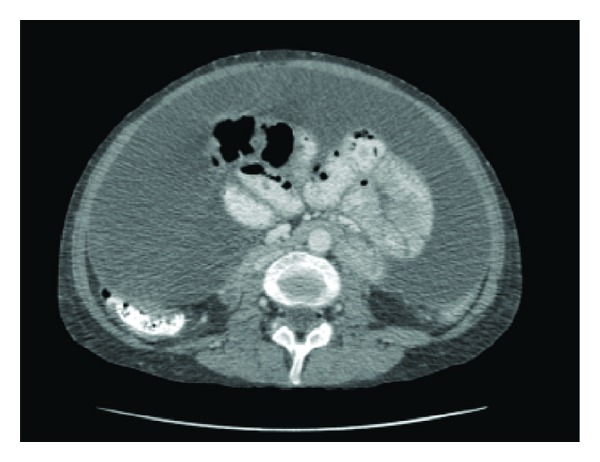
CT abdomen showing ascites and thickened peritoneum (June 2010).
